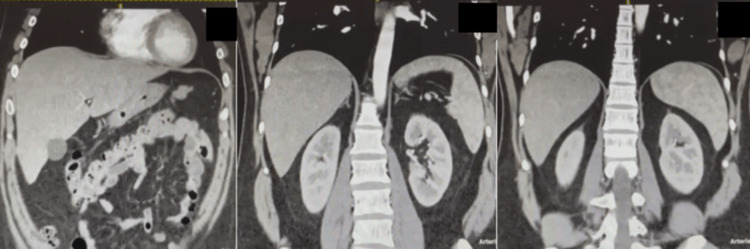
Representative images from the patient’s thoracic and abdominal CT scan. No cystic lesions are evident in the lungs, liver, spleen, or kidneys.
